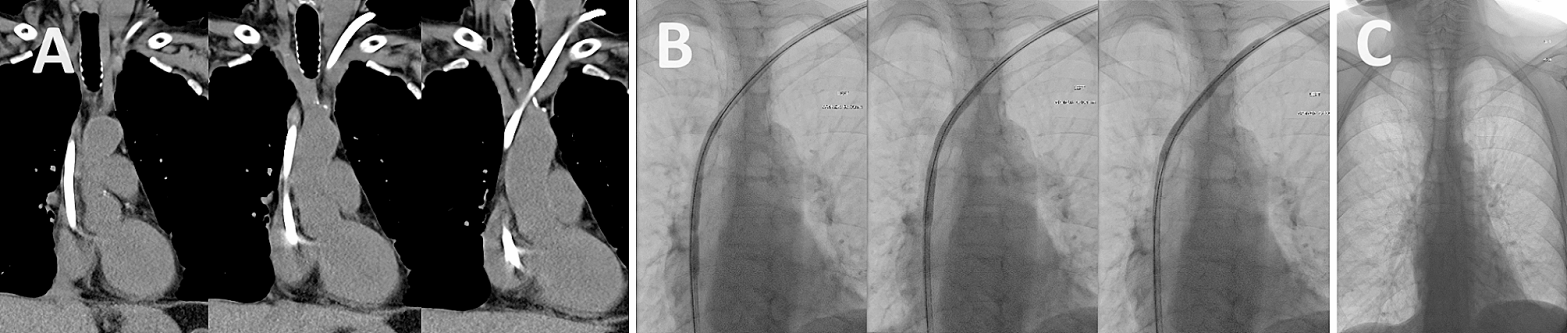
Peri-operative imaging of the incarcerated dialysis catheter. (A) CT pre-assessment, (B) Fluroscopic balloon dilation, (C) Post-operative chest x-ray
